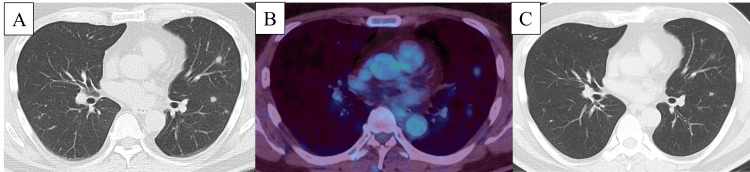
Radiological findings in Case 1. Chest CT showed multiple well-defined nodules in bilateral lung fields (A) and PET-CT showed fluorodeoxyglucose uptake in multiple lung nodules (B) and left hilum lymph nodes. Multiple nodules improved spontaneously (C). PET: positron emission tomography
